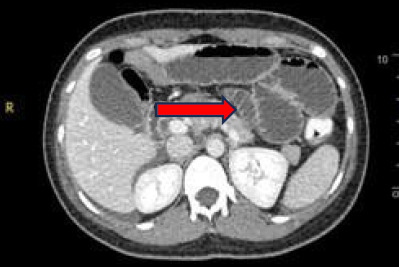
Computed tomography showed a dilated small bowel (arrow).
